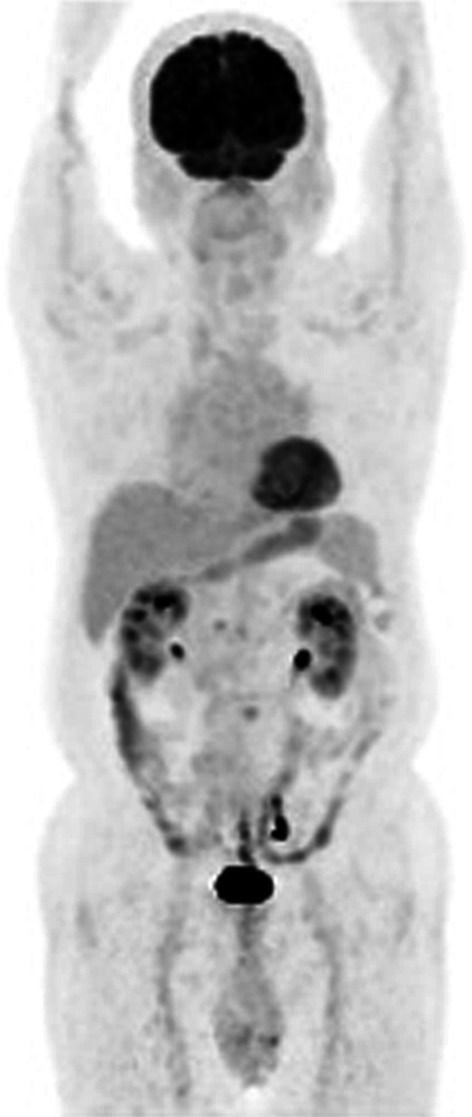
Image of F-18–2-fluoro-2-deoxy-D-glucose positron emission tomography computed tomography. No other affected organs were detected in the whole-body imaging postoperatively
